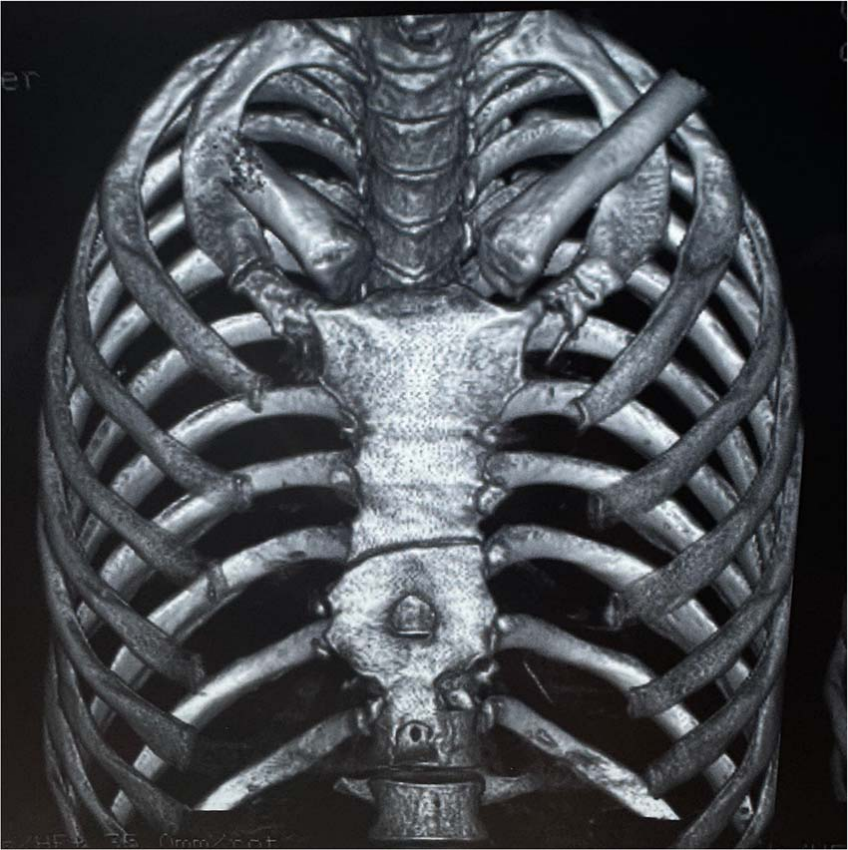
CT (3D reconstruction) revealing a non-displaced sternal fracture and the sternal foramen adjacent to the fracture site.
